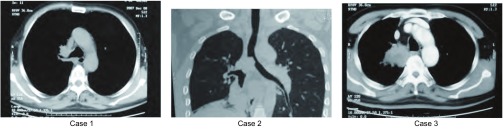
术前胸部CT示肿瘤累及隆突 The preoperative chest CT shows that carina is involved by lung carcinoma
